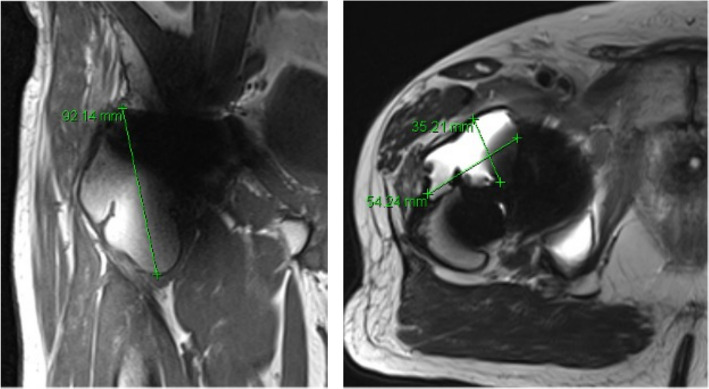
a MARS-MRI of the right hip, coronal plane, obtained 22 years post-operatively in a 72-year-old male patient. A cystic pseudotumor, measuring approximately 92 × 54 × 35 mm with a volume of about 90 mL, is observed adjacent to the joint. b MARS-MRI of the same right hip in an axial plane
